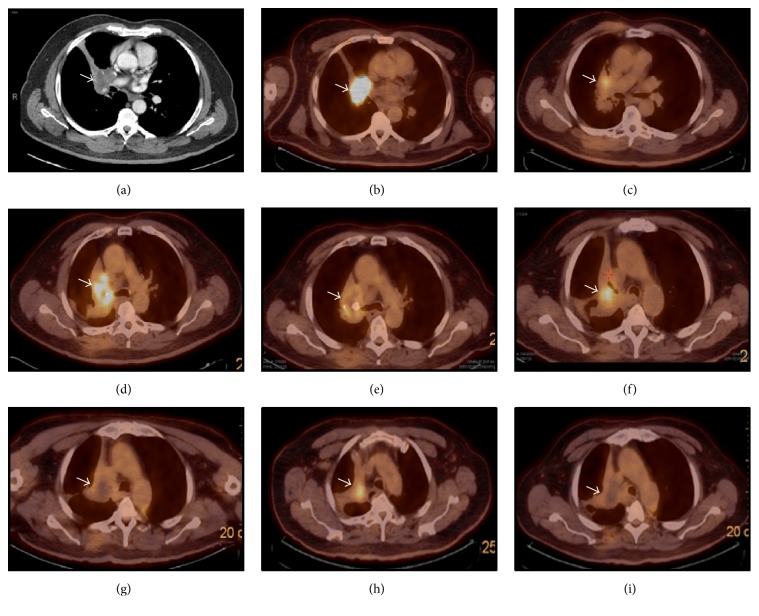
(a) CT scan showed right hilar mass (arrow) in July 2009. (b) PET scan confirmed FDG activity of the mass. (c) Partial response after radiation therapy (October 2009). (d) Extensive disease progression (May 2011). (e) Partial response after chemotherapy (December 2011). (f) Disease progression (March 2012). (g) Reduced metabolic activity after sunitinib (August 2013). (h) Disease progression (September 2014). (i) Complete disappearance of FDG activity with central necrosis after increased dose of sunitinib (February 2015).
